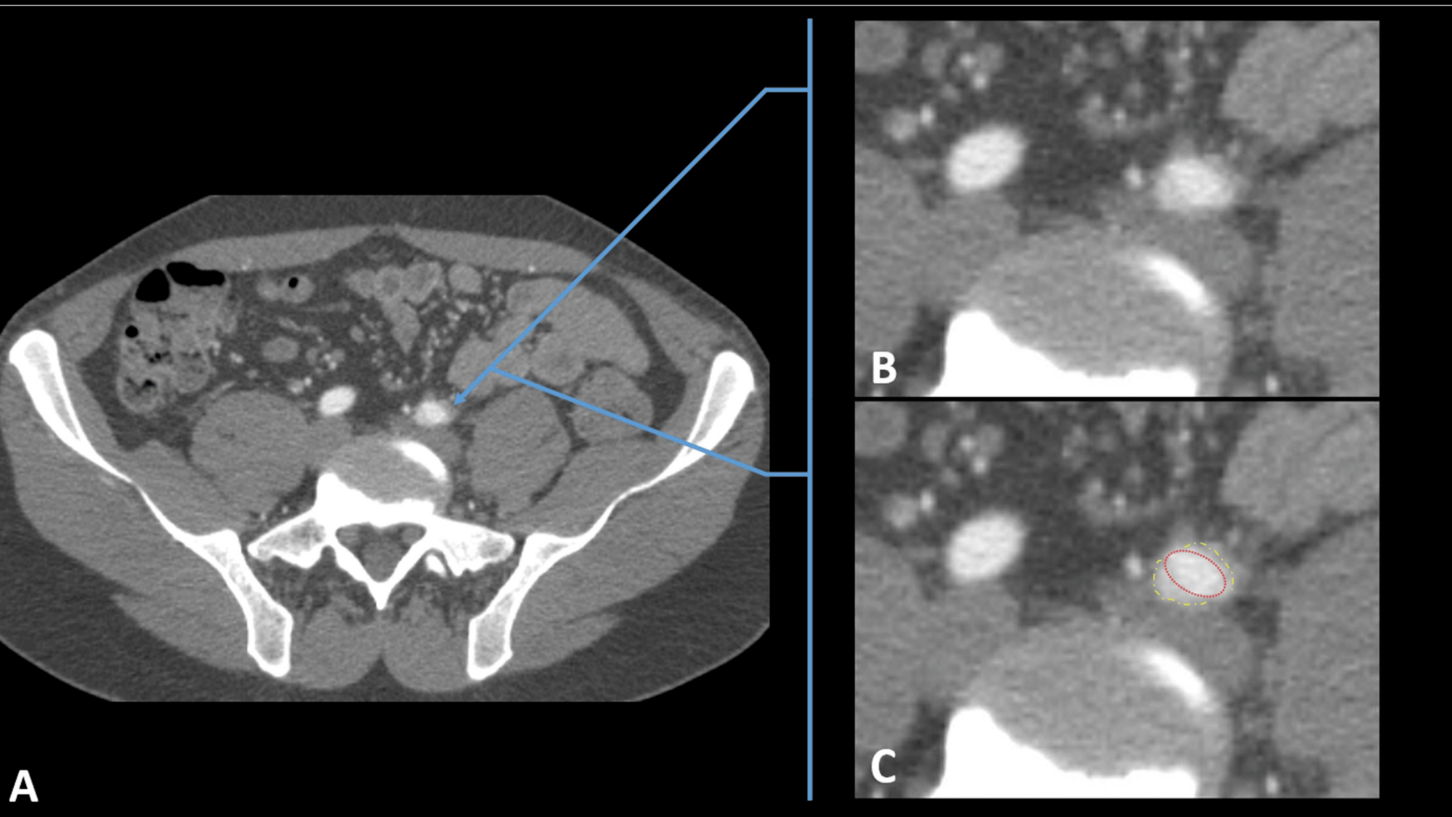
CT angiography of the pelvis in the axial plane Contrast-enhanced CT imaging of the pelvis in the early arterial phase provides evaluation of the arteries. Specifically, in this cross section, the common iliac artery (CIA) is shown bilaterally relative to surrounding pelvic viscera in a non-magnified view (A). (B and C) Cropped magnified axial images created from (A). (C) Annotations of the normal vessel (red perforated oval) versus the abnormal fibrotic change surrounding the vessel (yellow perforated oval).  The CIA on the left is pathologic, whereas the CIA on the right is normal (A-C).
